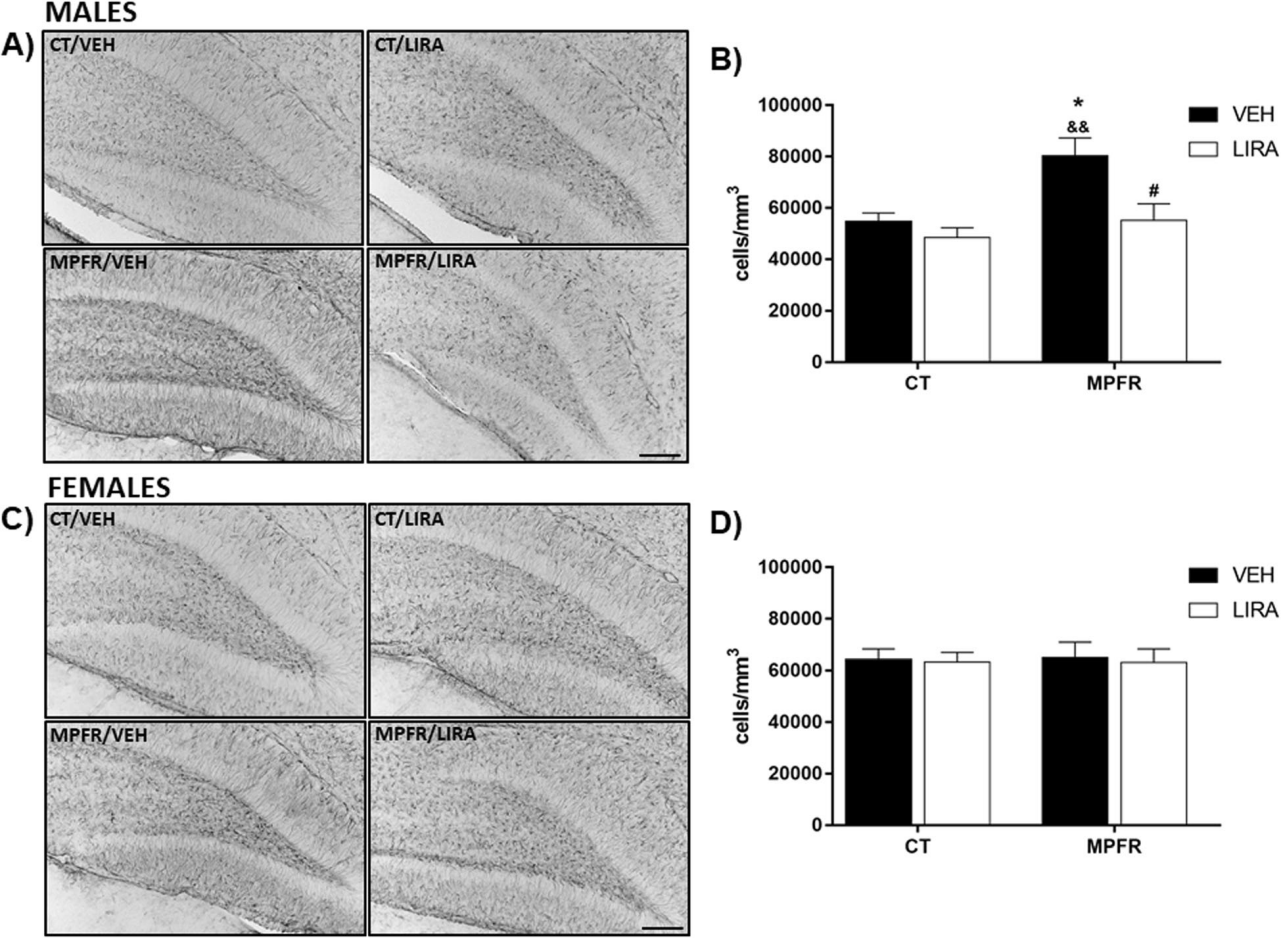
a Representative images of GFAP-immunoreactivity in the dentate gyrus of male pups. Scale bar, 30 μm. b Quantification of GFAP-1 immunoreactivity in the dentate gyrus in male pups. c Representative images of GFAP-immunoreactivity in the dentate gyrus of female pups. Scale bar, 45 μm. d Quantification of GFAP-1 immunoreactivity in the dentate gyrus in female pups. CT/VEH, control pups treated with vehicle; CT/LIRA, control pups treated with liraglutide; MPFR/VEH, food-restricted pups treated with vehicle; and MPFR/LIRA, food-restricted pups treated with liraglutide. Data are mean + SEM. (p* < 0.05 compared to CT/VEH; ^&^*p* < 0.05 compared to CT/LIRA; ^#^*p* < 0.05 compared to MPFR/VEH)
